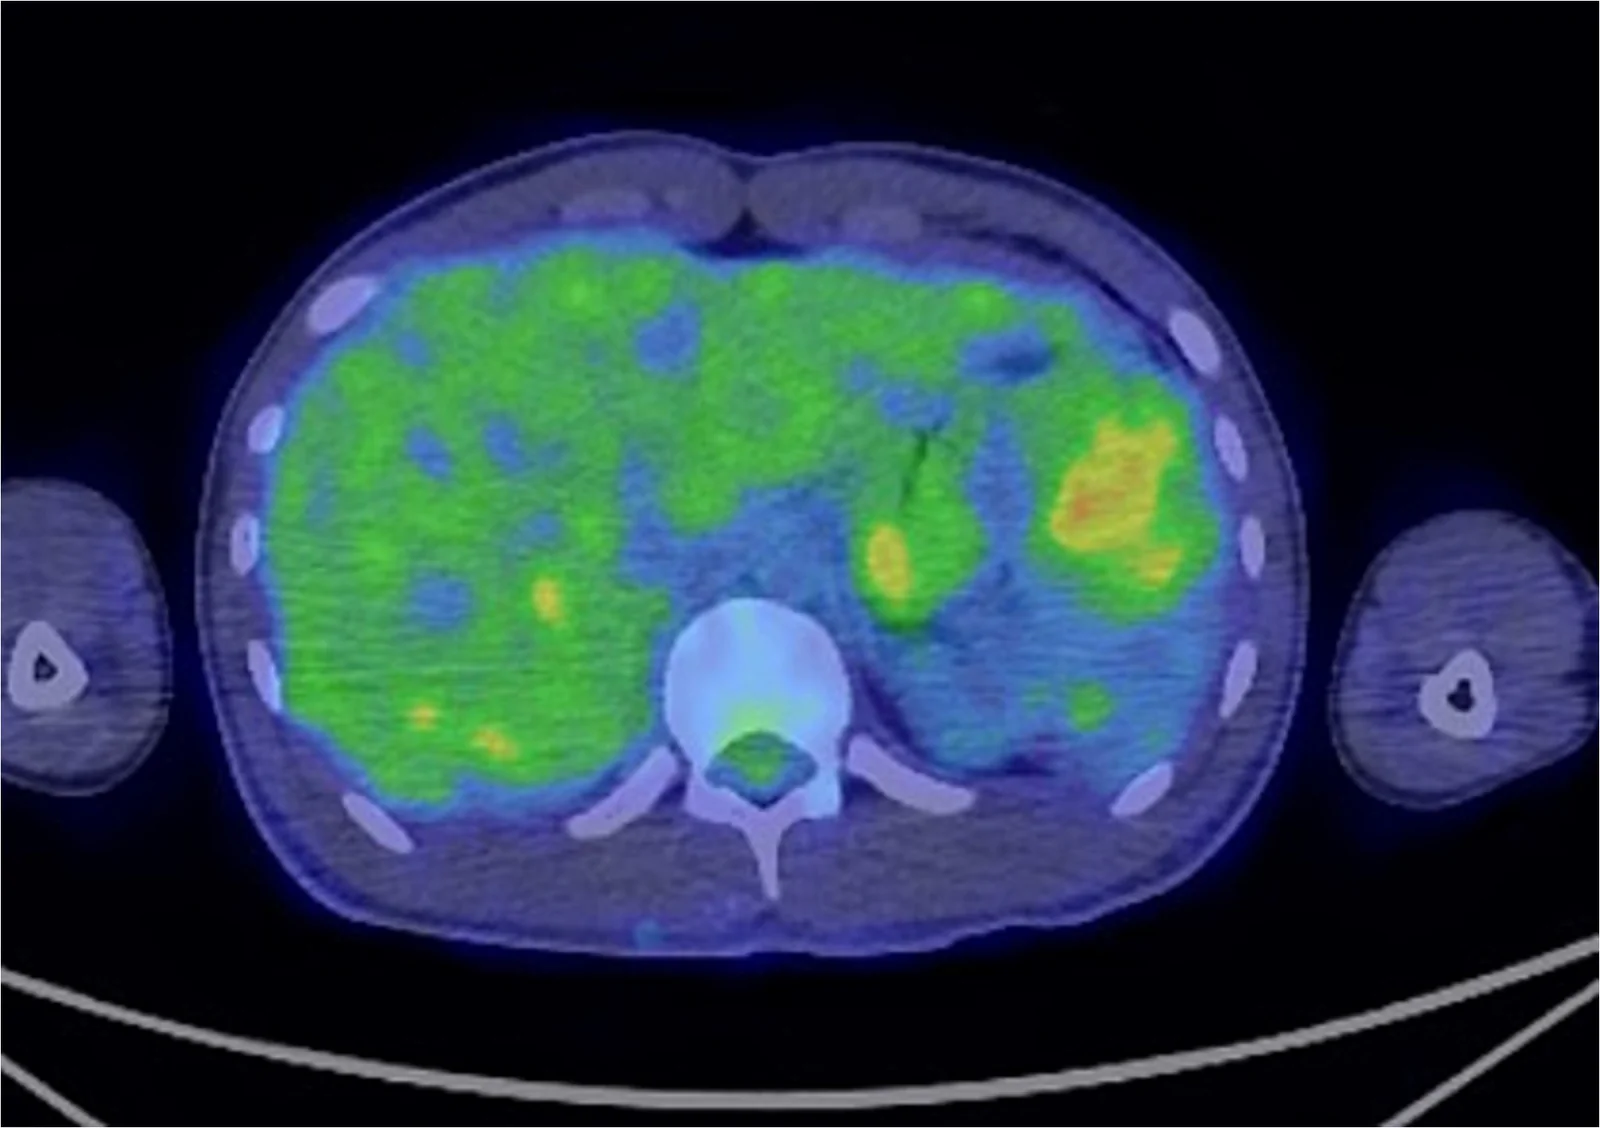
Positron emission tomography-computed tomography (PET-CT). PET-CT shows mild fluorodeoxyglucose uptake within the splenic lesion, with a maximum standardized uptake value (SUVmax) of 3.0. No intense accumulation suggestive of malignancy is observed.
